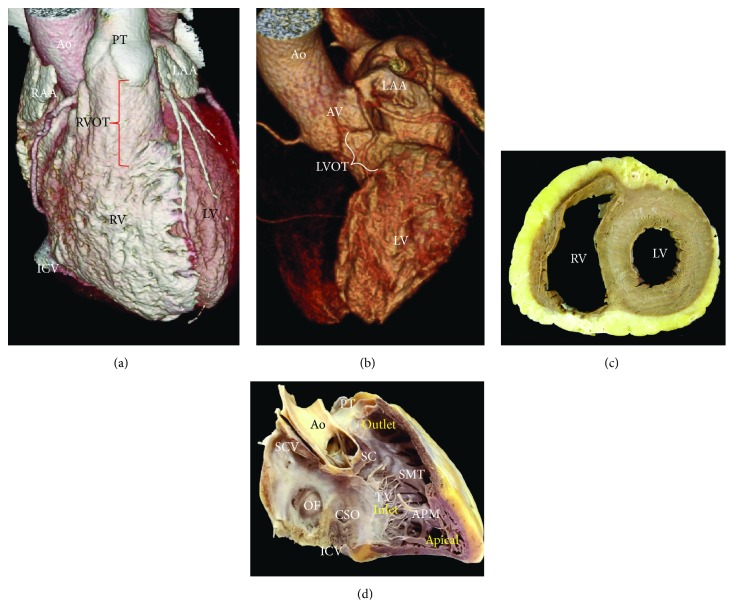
Right anterior views of volume rendered CT angiographies. (a) Right ventricle and (b) left ventricle. (c) The right ventricle is crescent-shaped in cross section. (d) This is a right lateral view showing the three components of the right ventricle and the characteristic muscle bundles as septomarginal trabeculation and supraventricular crest or ventriculoinfundibular fold. Ao = aorta, APM = anterior papillary muscle, AV = aortic valve, CSO = coronary sinus ostium, ICV = inferior cava vein, LAA = left atrial appendage, LV = left ventricle, LVOT = Left ventricle outflow tract, OF = oval fossa, PT = pulmonary trunk, RAA = right atrial appendage, RV = right ventricle, RVOT = right ventricle outflow tract, SC = supraventricular crest, SCV = superior cava vein, SMT = septomarginal trabeculation, and TV = tricuspid valve.
